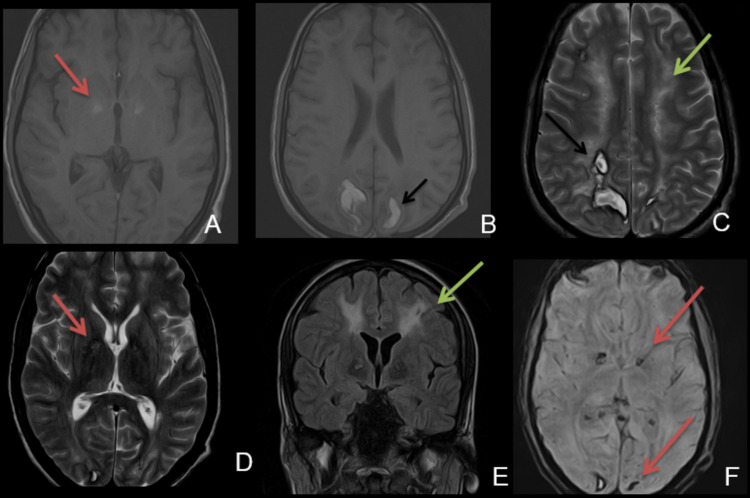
Multiplanar and multisequential MRI of the brain. A & B: T1 axial image showing T1 hyperintense signal in the globus pallidus (red arrow, typical finding of CO poisoning) and hemorrhage in the posterior parietal regions involving the gray-white matter junction and watershed areas (black arrow). C: T2 axial image showing abnormal T2 hyperintense signals in the subcortical white matter, centrum semiovale (green arrow), and hemorrhage in the posterior parietal regions (black arrow). D: T2 axial image showing T2 hyperintense signal in the globus pallidus (red arrow). E: FLAIR coronal image redemonstrating abnormal white matter signals. F: SWI showing signal dropout in the bilateral globus pallidus and posterior occipital region, more on the right (red arrow). CO: carbon monoxide; FLAIR: fluid-attenuated inversion recovery; MRI: magnetic resonance imaging; SWI: susceptibility-weighted imaging
